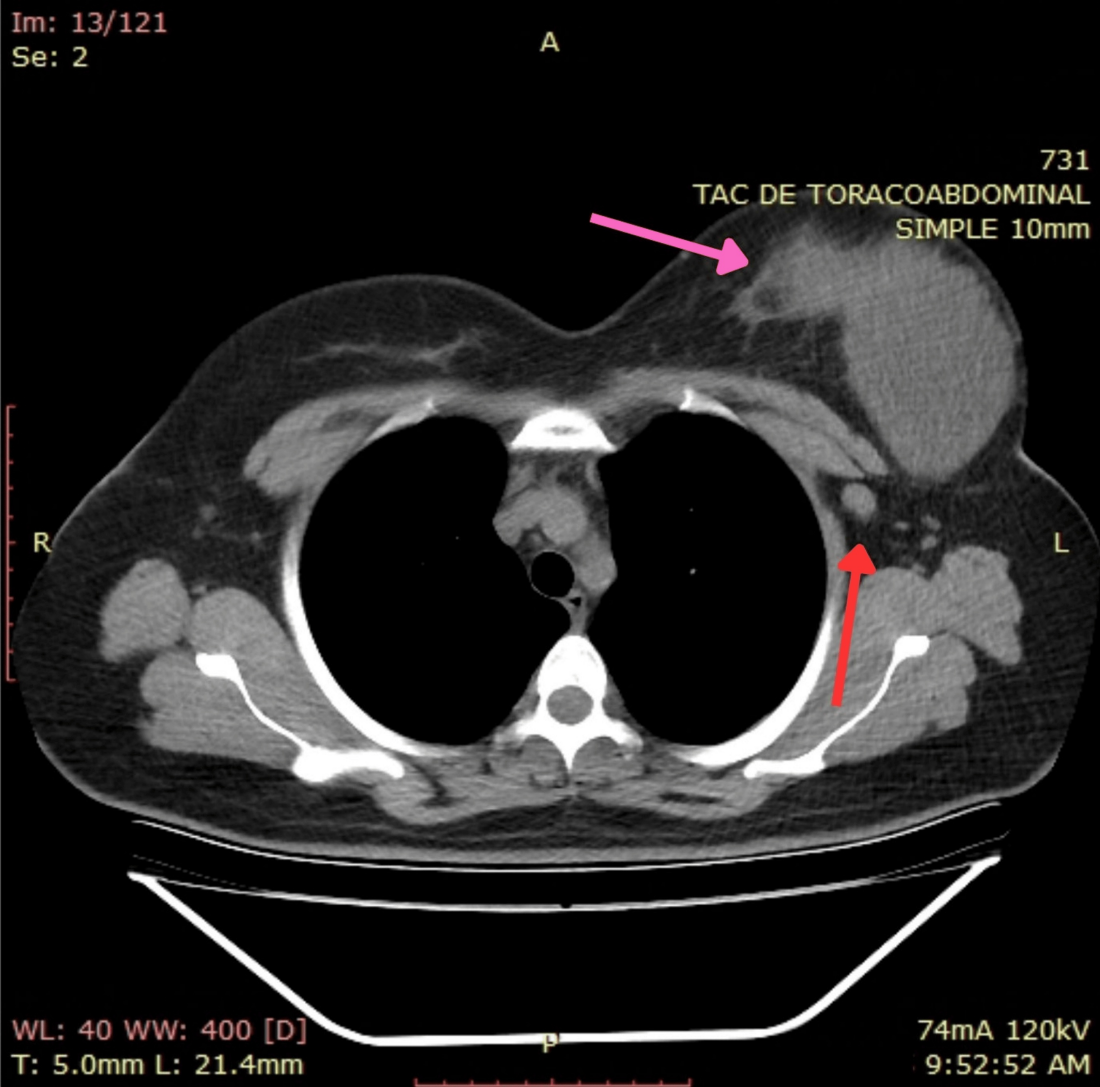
Thoracic CT scan showing a left breast mass, having contact with the skin and ipsilateral extension to the left axillary region Red arrow: Extension to left axillary region Pink arrow: Left breast mass having contact with skin
